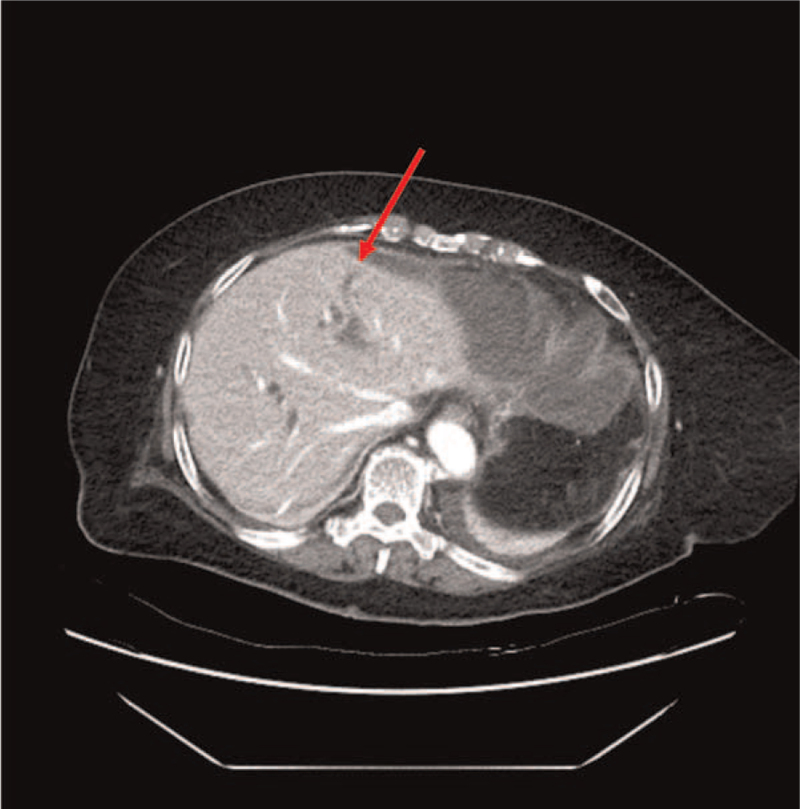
Computed tomography shows large loculated fluid collection in perihepatic space. Red arrow indicates the point where bile leakage was found during surgery.
